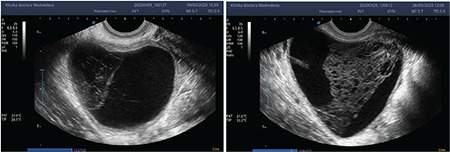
Typical sonographic features of a corpus luteum cyst with hemorrhagic content
